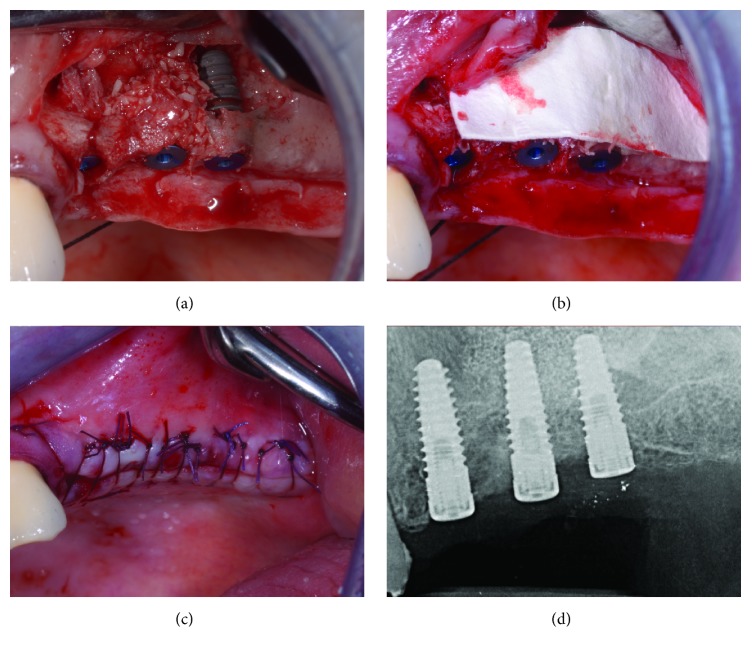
After partially filling the grafting site, implants are being placed with the aid of the surgical guide (a) and filling is complete (b). Implants are left submerged (c), and an intraoral control radiograph is collected (d).
